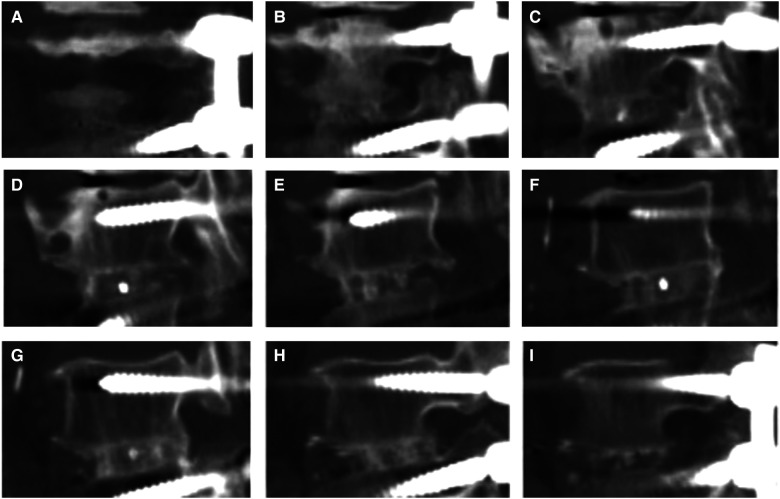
For lumbar 4-5 TLIF, the decompression side is on the left, and 2D CT reconstruction in our hospital was scanned from right to left. From (A–C), the opposite side of decompression was observed continuously from the first image until the appearance of the cage marker. (D–F) is the front, inside and back areas of the cage, and the marker was observe from one side of the cage to the other side. (G–I) showed the decompression area, and the last image was observed after the marker on the other side of cage disappeared.
